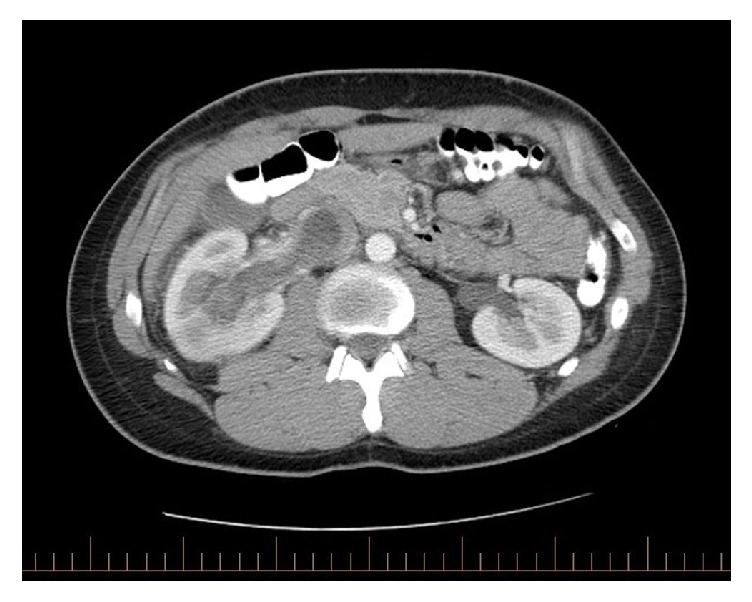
Axial, contrast-enhanced CT demonstrates a centrally located lobulated mass invading the renal vein and extending into the lumen of the inferior vena cava.
